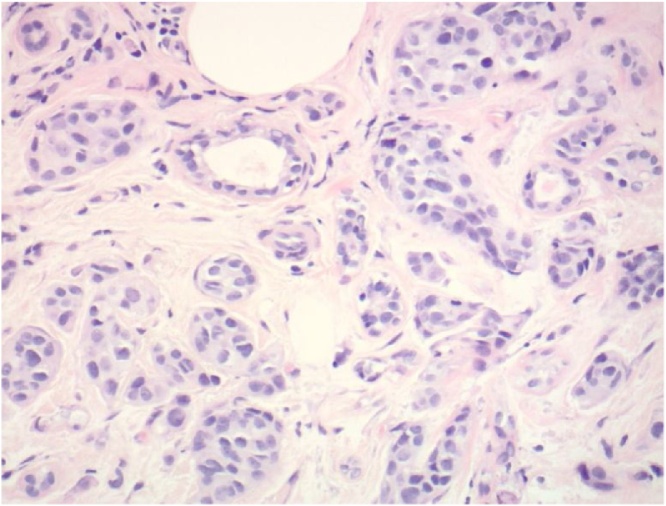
A radiographically guided core biopsy of a spiculated mass in the left breast shows intermediate grade invasive mammary ductal carcinoma (left breast, H & E, 200× original magnification).
